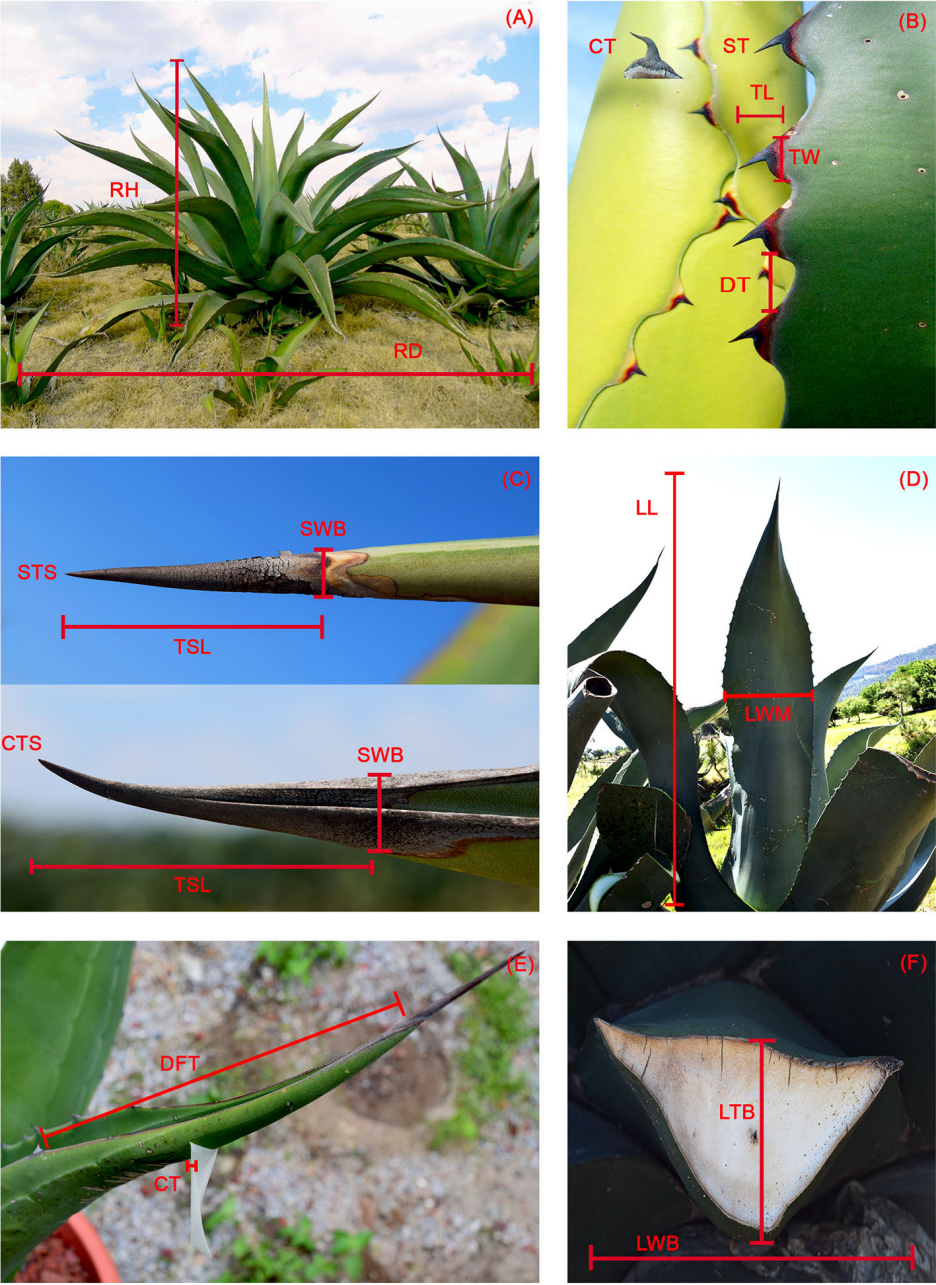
Morphological characters. (A) RH, rosette height; RD, rosette diameter. (B) CT, curved teeth; ST, straight teeth; TL, tooth length; TW, tooth width; DT, distance between teeth. (C) STS, straight terminal spine; CTS, curved terminal spine; SWB, terminal spine, width at base; TSL, terminal spine length. (D) LL, leaf length; LWM, leaf, width at mid length. (E) DFT, distance to first tooth; CT, cuticle thickness. (F) LTB, leaf thickness at base; LWB, Leaf width at base.
